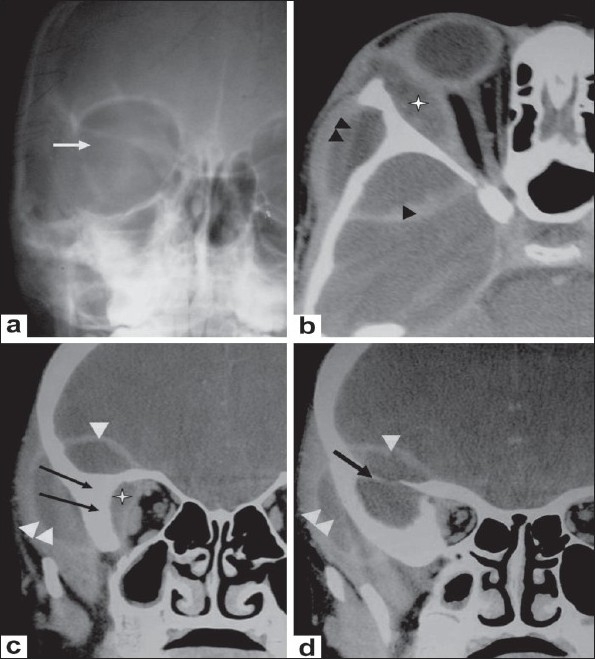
A 10-year-old boy presented with unilateral, painless, nonpulsatile proptosis of the right eye with low-grade fever. Caldwell view radiograph (a) shows loss of definition and fuzziness of the greater wing of the sphenoid on the right side (arrow). Axial (b) and coronal (c,d) contrast-enhanced CT scans of the orbit, show a rightsided extraconal and lacrimal abscess (asterisk in b,c), an intracranial extradural abscess (arrowhead), and an abscess in the infratemporal fossa region (double arrowhead). Irregularity and sclerosis of the sphenoid and zygomatic bones (arrow in c,d) is also seen.
